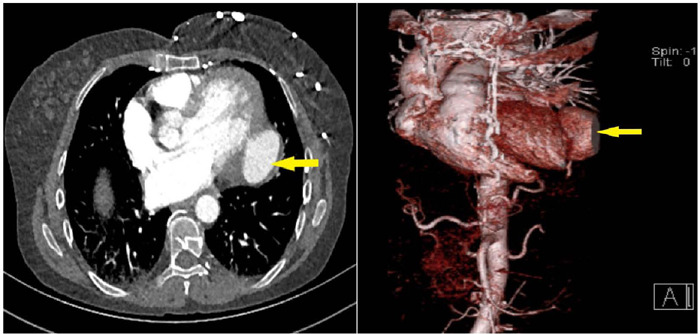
Computed tomography angiography image of left ventricular aneurysm
